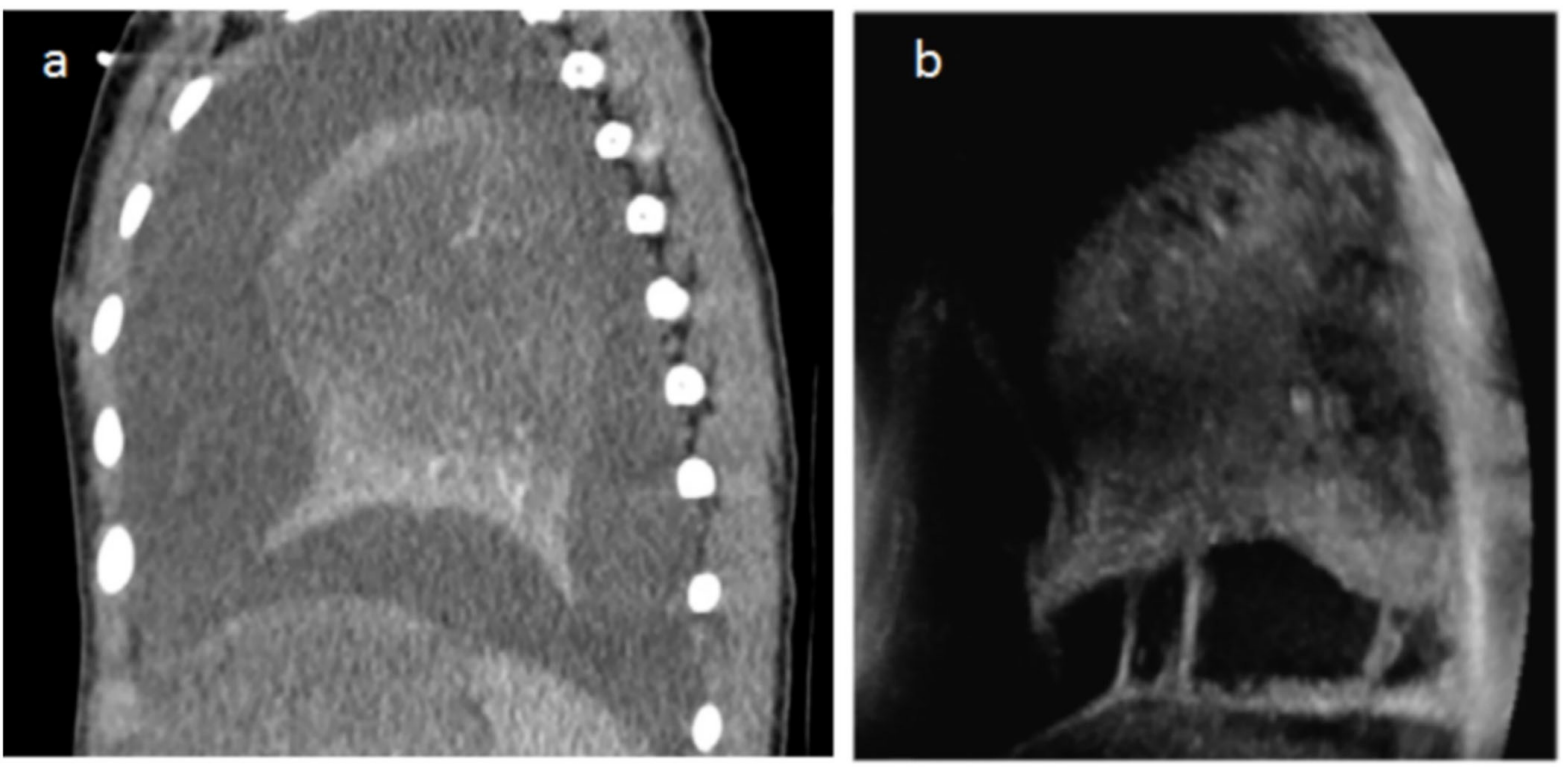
(a) Chest CT with contrast agent injection in a 2.5-year-old child with 39°C fever, cough, and breathing difficulties. Sagittal reconstructions show a heterogeneous lung with unenhanced parenchyma corresponding to necrosis and a large pleural effusion. The US performed the same day also shows heterogeneity and hypodensity of the parenchyma in the necrotic zones (same as on CT) and pleural effusion. The periphery of the lower lobe is spared in a similar way on LUS and CT. In addition, US demonstrates bands of fibrin within the effusion, not visible on CT (b).
